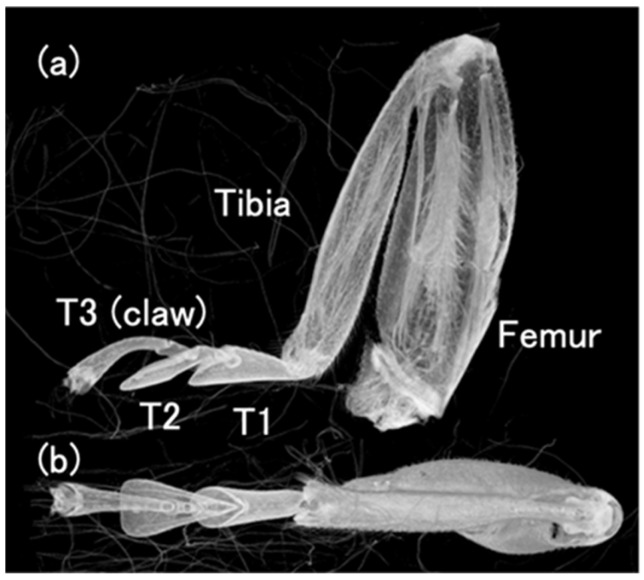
Left foreleg of ladybird beetle observed by micro-X-ray CT. (a) Side view and (b) top view. T3; Tarsal claw, T1 and T2; Tarsus.
